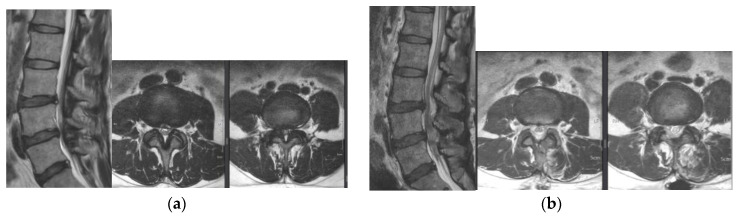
Pre-operative (a) and post-operative (b) MR images of a 76-year-old female patient with L3–4–5 spinal stenosis who underwent BESS surgery.
